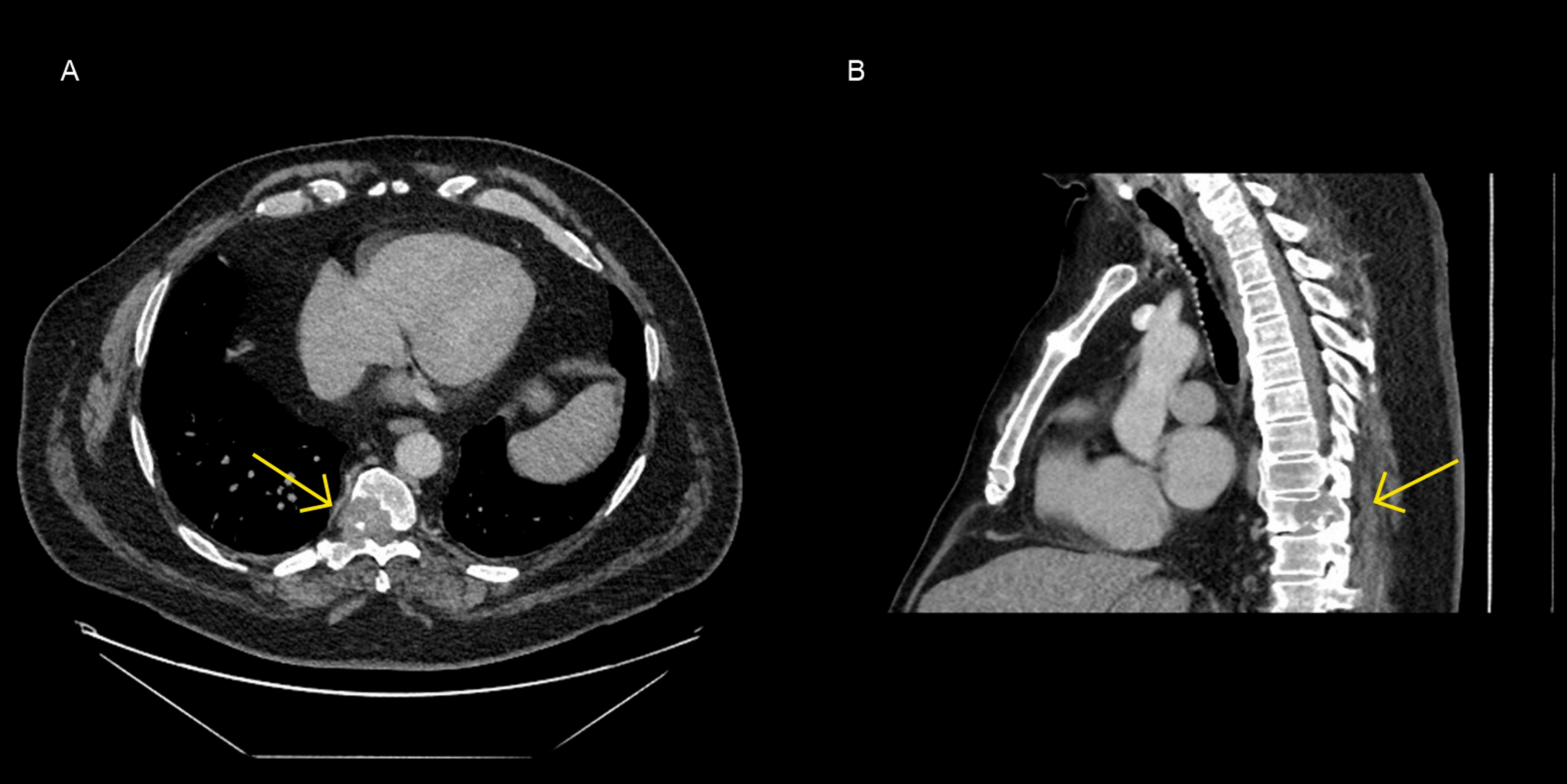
Contrast-enhanced CT of the thorax. (A) Axial and (B) Sagittal CT slices demonstrating a T9 destructive bone lesion with soft tissue involvement causing spinal stenosis (yellow arrows).
